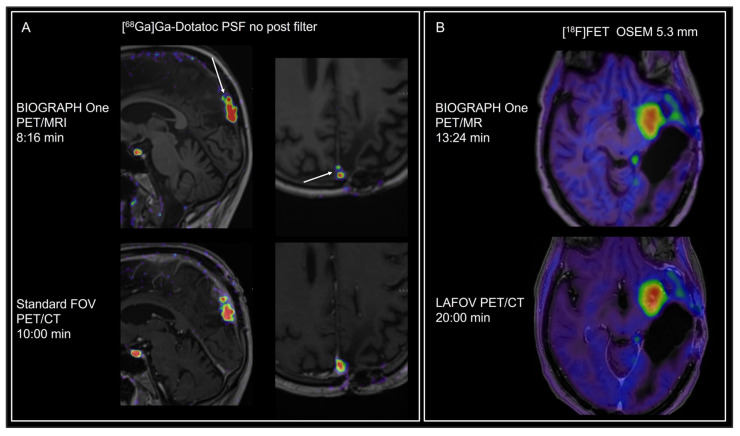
Short-duration imaging of brain tumors. (A) [^68^Ga]Ga-DOTATOC imaging in a patient with residual meningioma WHO grade 2 in the sagittal sinus. Images are scaled to the same SUV range. Tumor SUV values and tumor volume (SUV > 2.5) deviated by <5%. Note the better spatial separation of the smaller superior tumor component (white arrows) on BIOGRAPH One PET/MRI compared to standard FOV PET/CT despite the shorter acquisition time, although later imaging may also have improved the signal-to-noise ratio. (B) [^18^F]FET imaging in glioma. Patient with multifocal progressive isocitrate dehydrogenase–mutant astrocytoma (WHO grade 3). Total tumor-to-background ratios (TBR) and metabolically active tumor volume (TBR > 1.6) differed by less than 3%. Comparable image quality was achieved with clear delineation of multiple tumor components despite a shorter acquisition time. Long axial field-of-view (LAFOV) PET/CT is shown fused with prior contrast-enhanced T1-weighted MRI.
